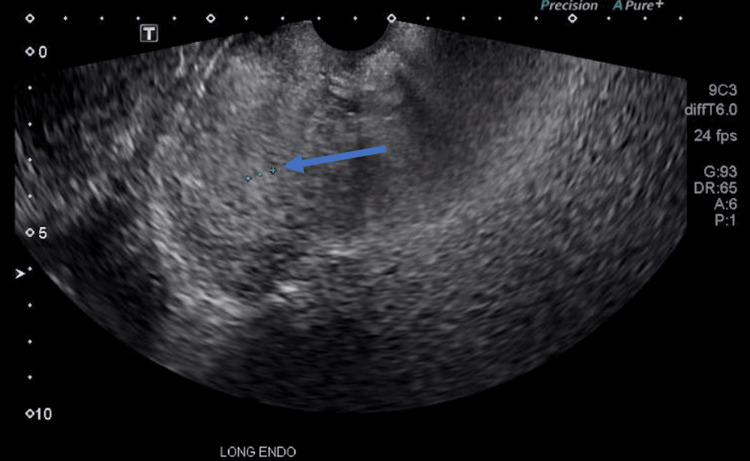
Transvaginal ultrasound demonstrating the long endometrial view of the uterus with an unremarkable endometrium measuring 7 mm in thickness (arrow).
